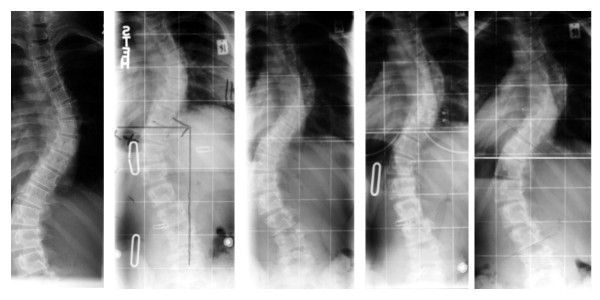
Radiological history of the patient. From left (x-ray taken outside our centre before start of treatment) to right the radiological course of the patient can be seen (last picture after 27 months on the right). A progression of the curve is visible.
